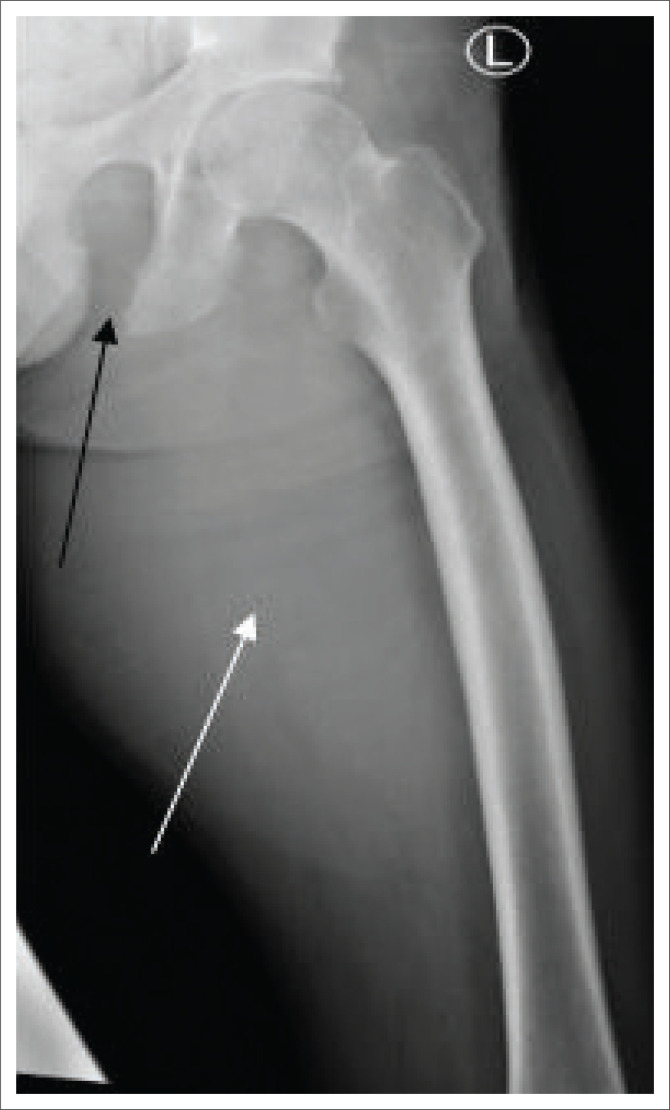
Left frontal thigh radiograph. Left thigh prominent soft tissue swelling (white arrow) associated with erosion of the left inferior pubic ramus (black arrow).
